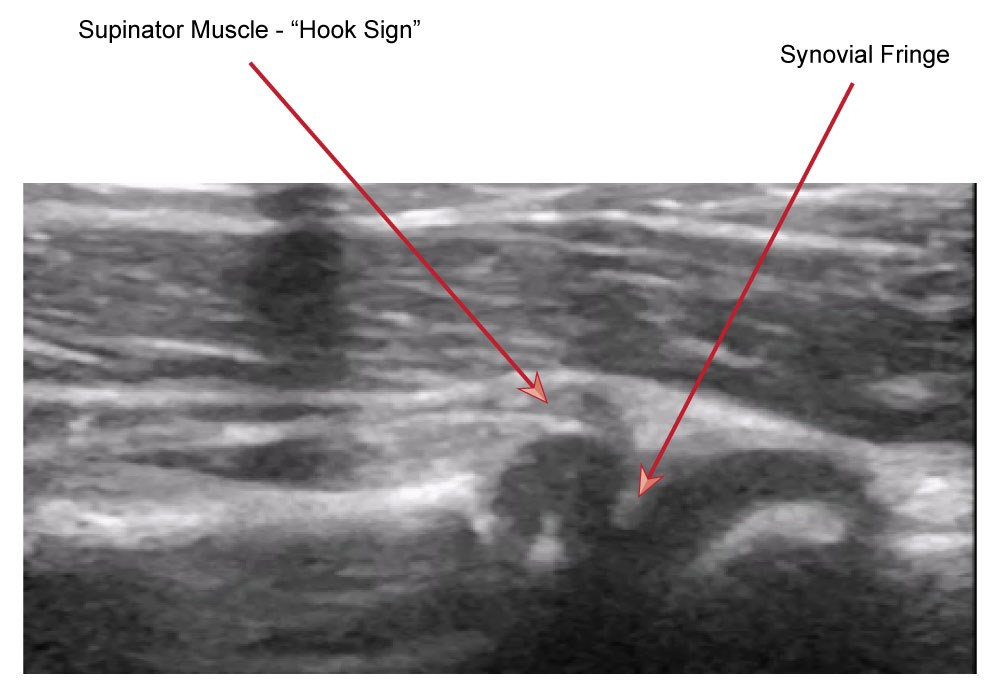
The sonographic signs consistent with pulled elbow.
